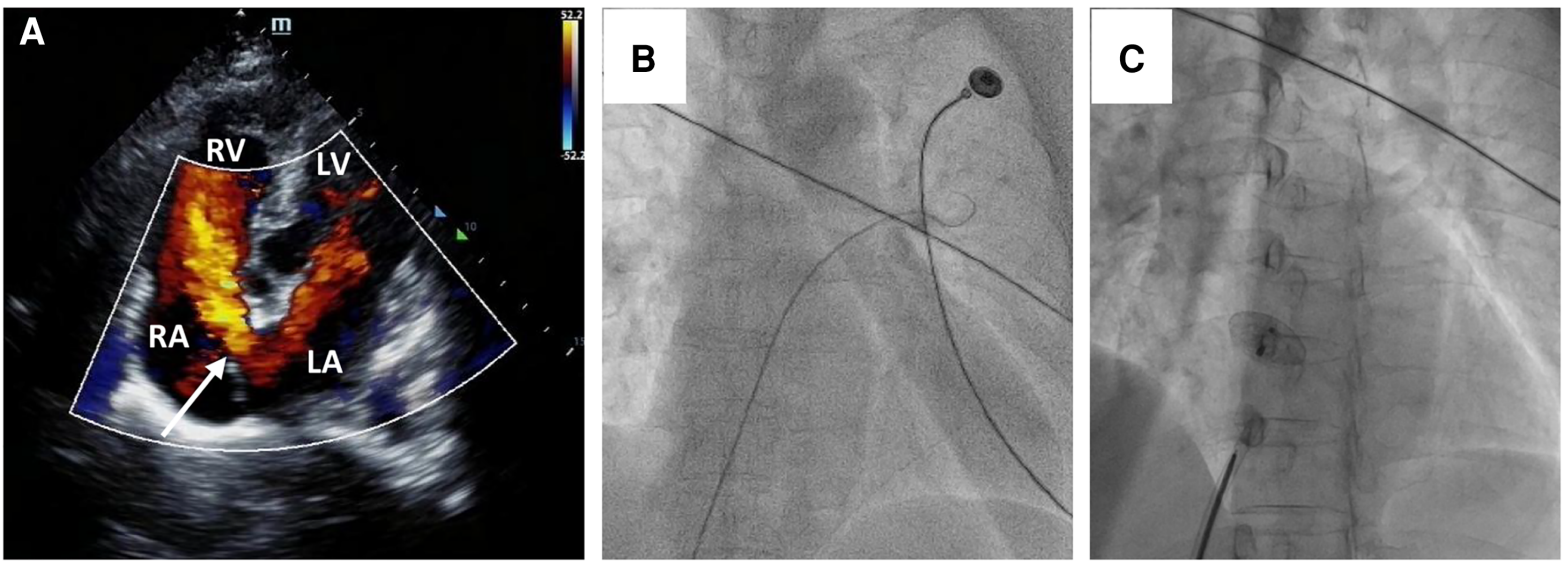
Secundum-type ASD finding and percutaneous secundum-type ASD closure. Transthoracic echocardiography revealed about 22 mm ASD (white arrow) with shunt flow from left atrium to right atrium (A) The intraoperative fluoroscopy showed that the guidewire passed through the inferior vena cava and the right atrium sequentially, and then through the defect site to the left atrium (B); a 24 mm ASO device was successfully deployed in the ASD (C).
